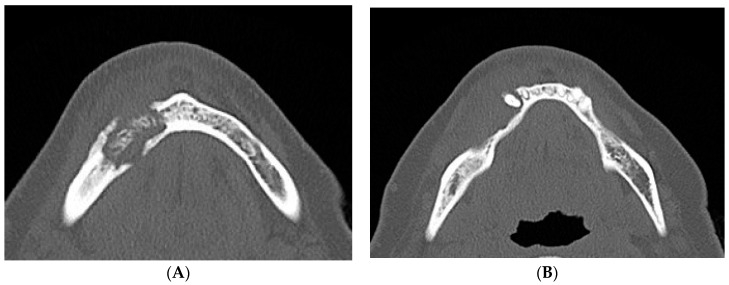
(A,B) Computed tomography (CT) scan dated February 2015, which showed a large sequestrum in right mandible along with erosion of the buccal and lingual cortical plates.
